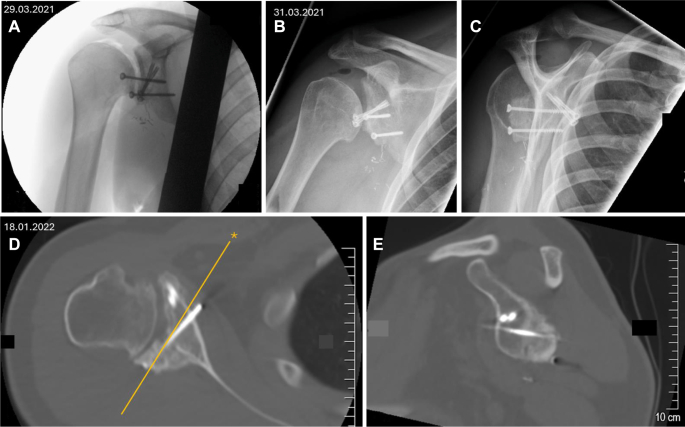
(A) Intraoperative fluoroscopy of the coracoid bone transposition in the Latarjet procedure to treat ventral shoulder dislocation. (B and C) Post-operative x-ray of the right shoulder two days after coracoid bone transposition and one year after posterior vascularized scapular bone flap. (D and E) 2-year follow-up: axial CT image demonstrating vital posterior and anterior bone flaps. *CT*, computer tomography.
